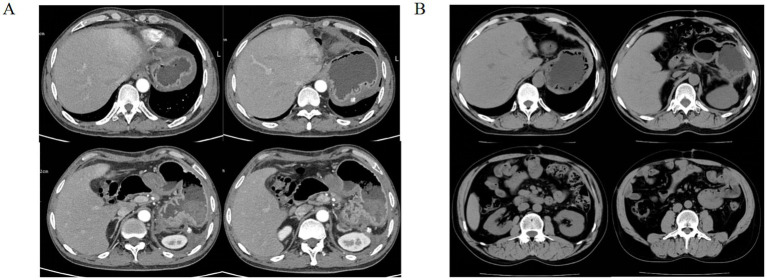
(A) Upper abdominal CT at 1 month after discharge demonstrated that the surgical drain could be safely removed. (B) Upper abdominal CT at 1 year after discharge revealed no obvious abnormality in the operative field.
